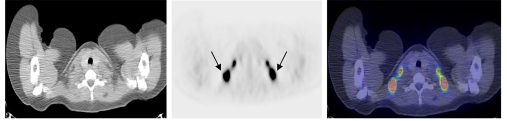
From left to right - axial CT image, PET image and fused PET CT image at the same level showing areas of intense uptake fat in the supraclavicular regions (arrows). The PET/CT fused images facilitated differentiation between fat and pathological tissue [Image courtesy of Centre for Molecular Imaging, Peter MacCallum Cancer Centre, East Melbourne, Victoria, Australia].
